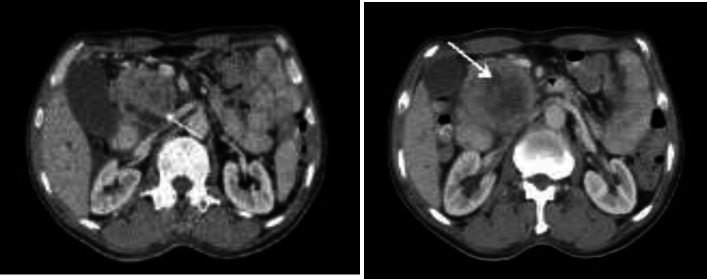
Computed tomography image showing an 8.8 x1.6.8 x1 cm mass in the head of pancreas
